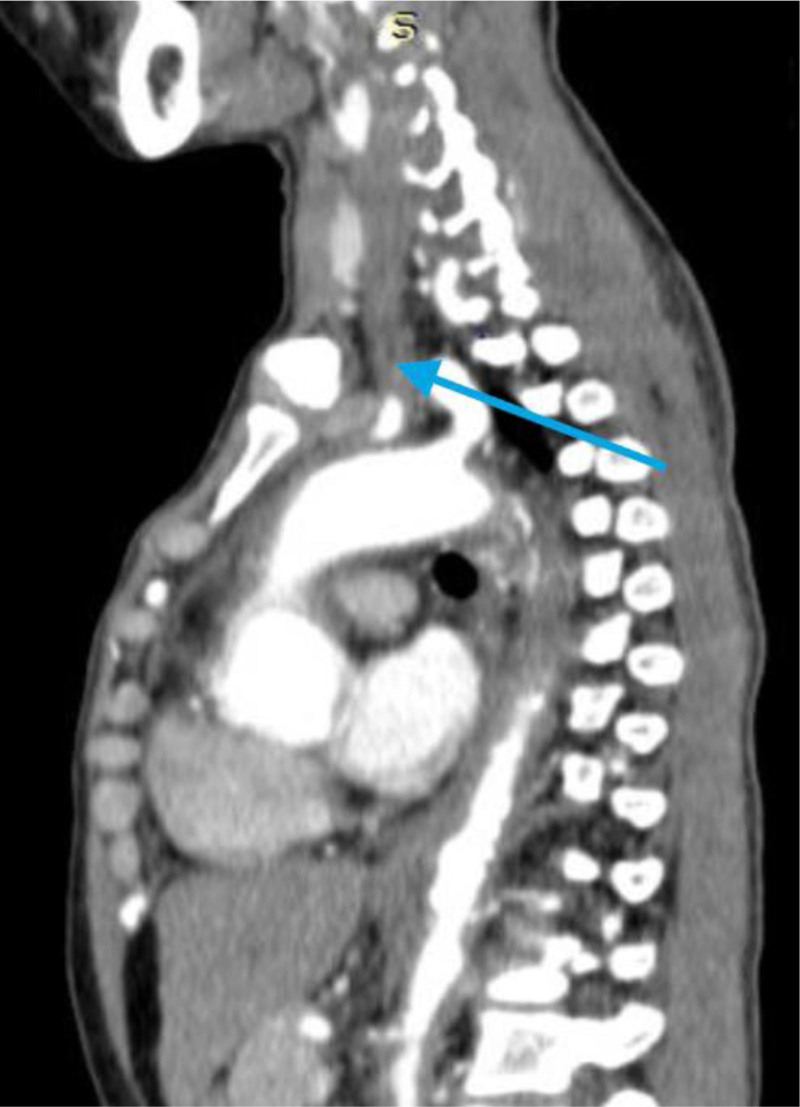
Post-contrast CT scan: thickened, stenosed, occluded, and thrombosed left common carotid artery (blue arrow). Descending thoracic aorta and abdominal aorta wall thickening and luminal narrowing were visualized. CT scan = computed tomography scan.
